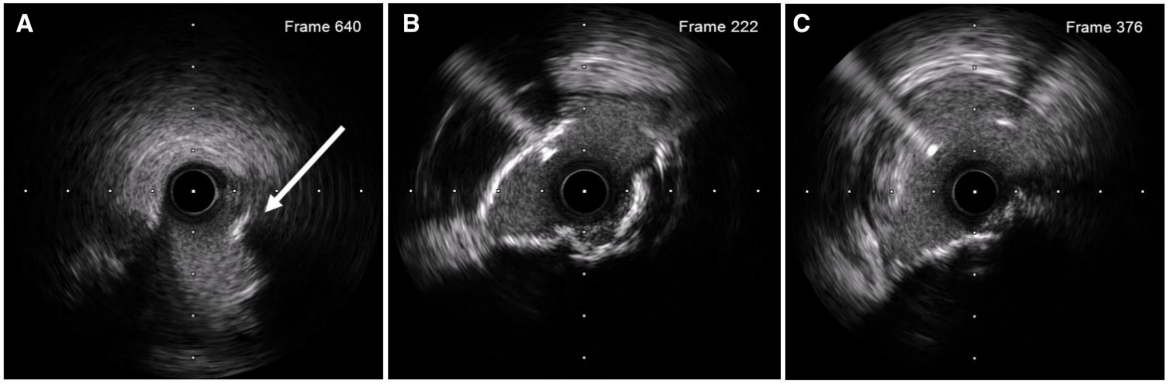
Intravascular ultrasound of the circumflex confirmed a calcified nodule (white arrow) with a mild thrombotic lesion with a minimal luminal area of 1.2 mm^2^ (*A*). Ostial disease of the left anterior descending with 270° arc of calcification and minimal luminal area of 4.8 mm^2^ (*B*) and the minimal luminal area of the left main stem was 5.9 mm^2^ (*C*).
